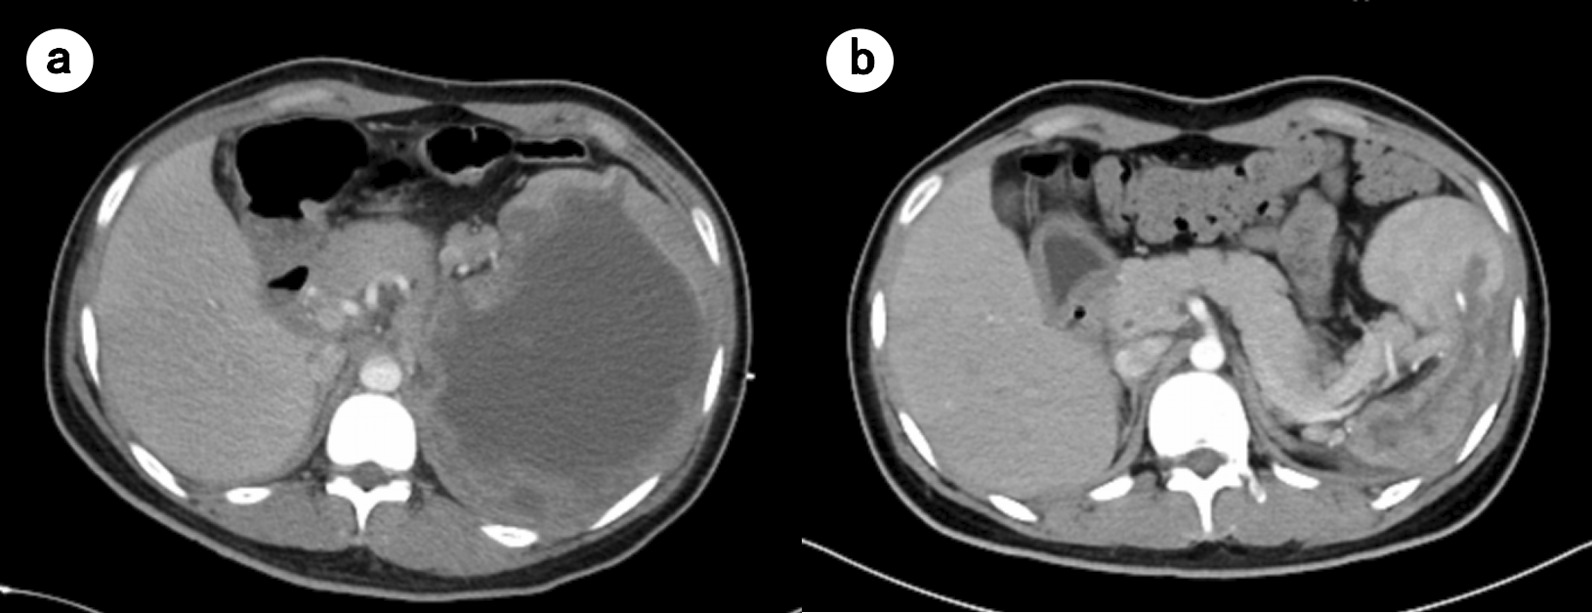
Abdominal CT scan on admission showed giant cystic lesion within the spleen (a). A follow-up abdominal CT taken 42 days later exhibited that the abscess had significantly decreased (b)
